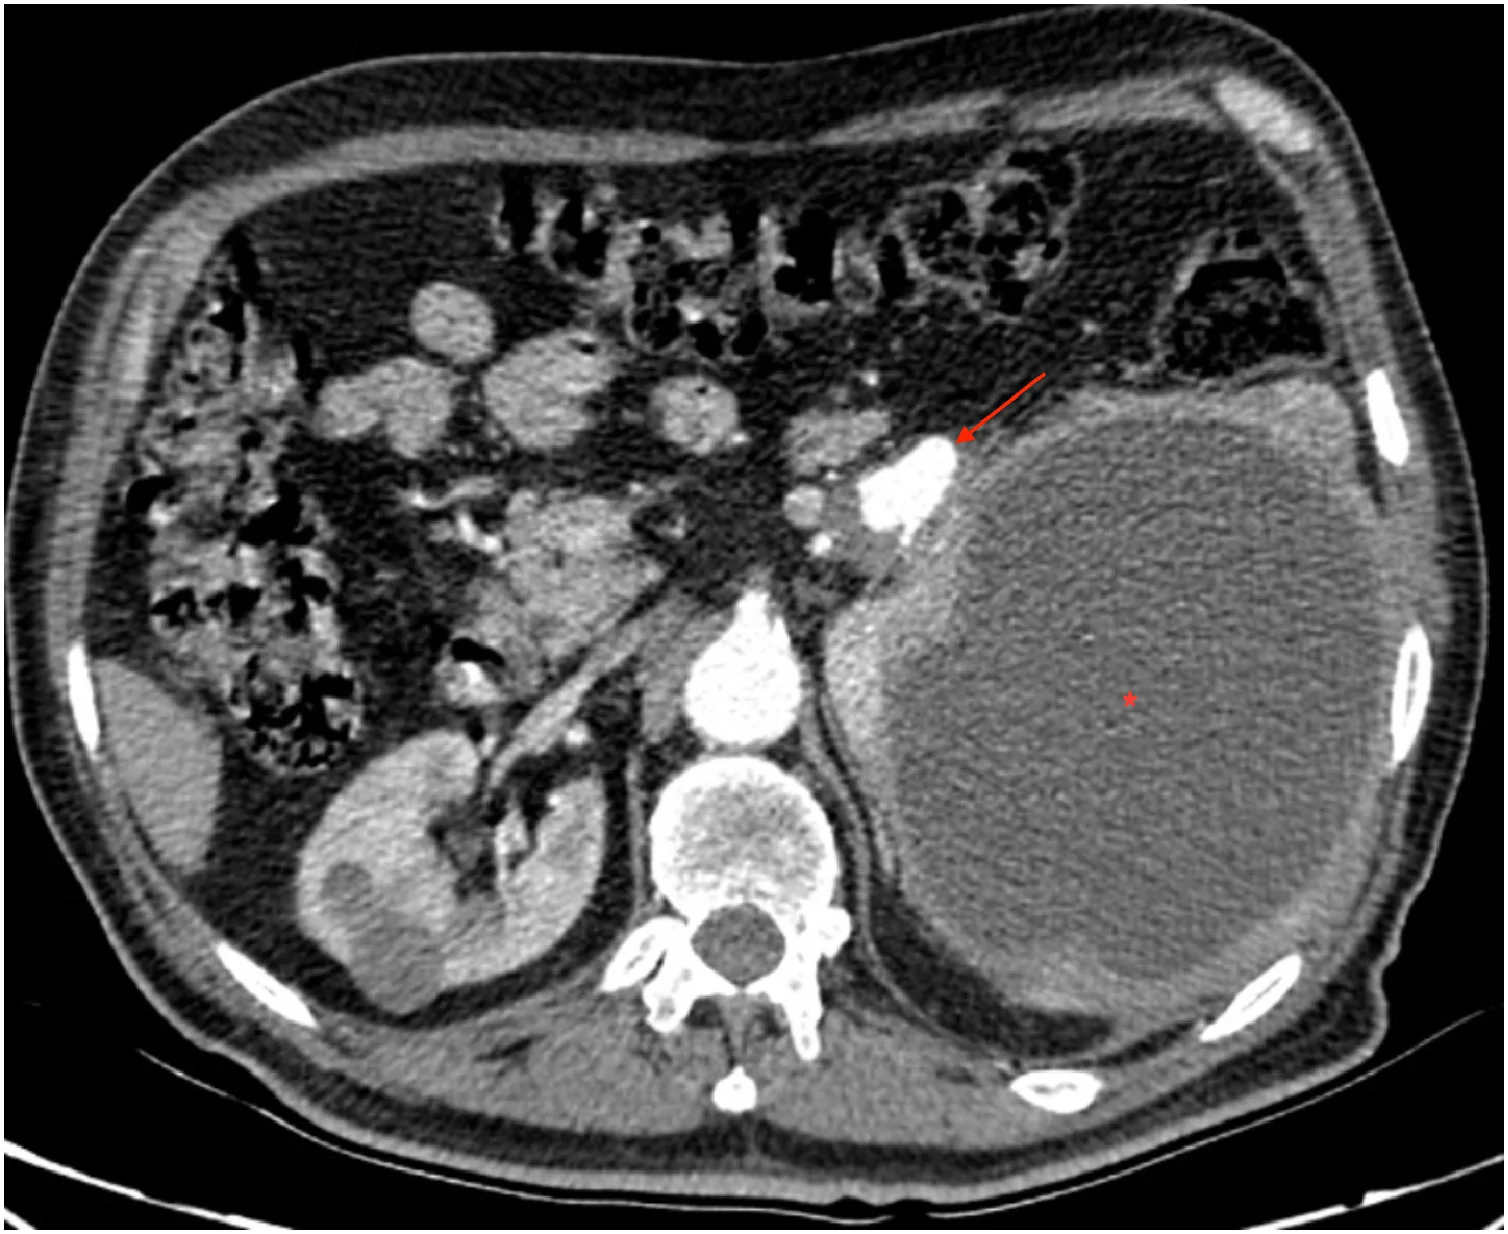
Axial contrast-enhanced CT image, arterial phase, demonstrating a large cystic splenic lesion (asterisk) with associated intralesional inflammatory changes consistent with abscess formation. Arrow indicates a focal vascular structure within the lesion suggestive of a splenic artery pseudoaneurysm.
